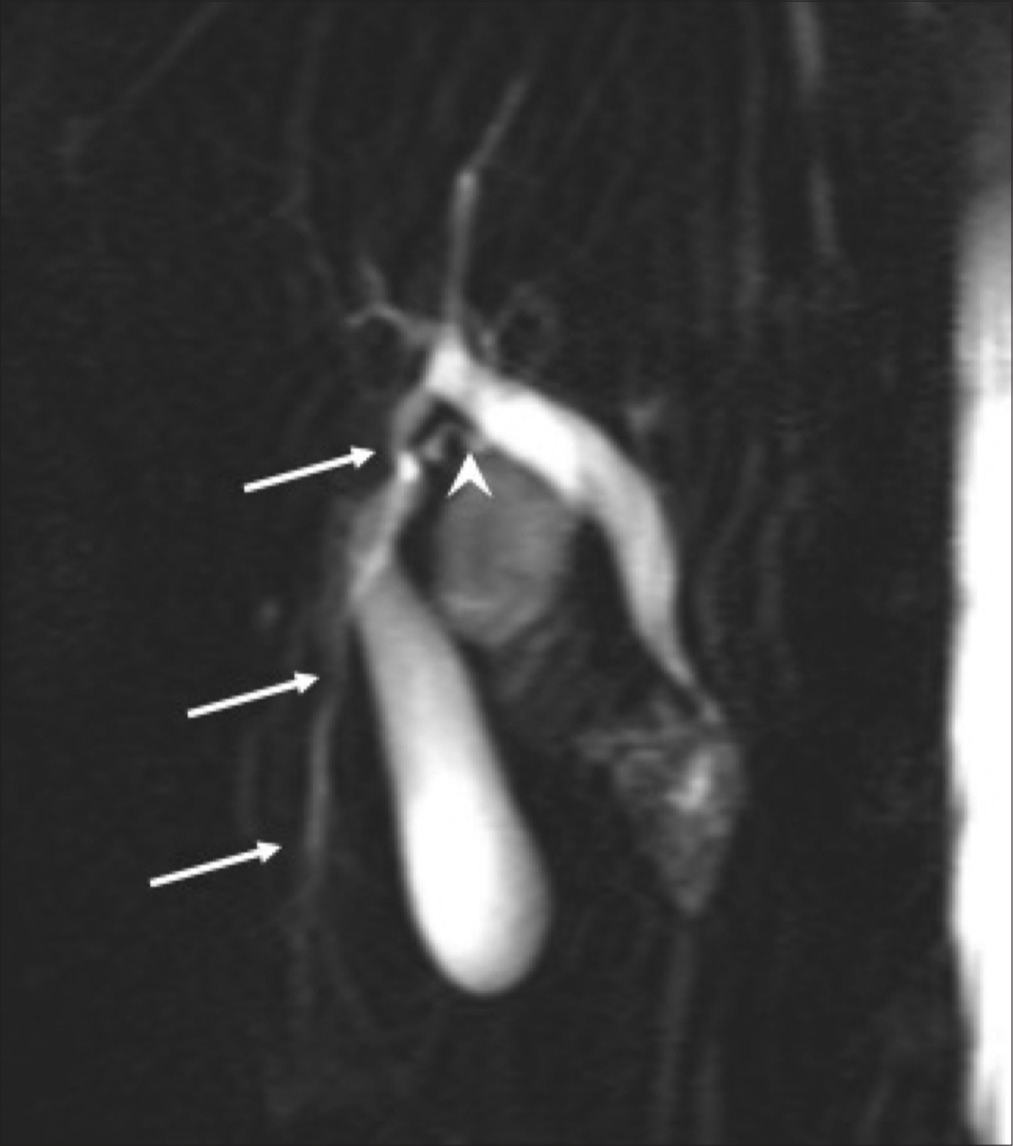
MRI cholangiopancreatography image shows the accessory duct draining into the common hepatic duct (arrows). Notice also a separate, tortuous, cystic duct opening into the bile duct (arrowhead).
